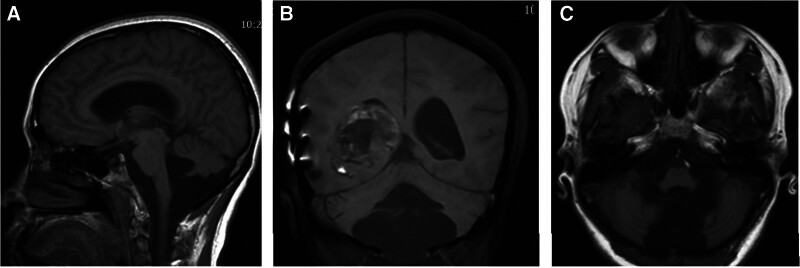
Posterior fossa MRI sections showing Dandy-Walker variant. Imaging of the posterior fossa showing increased retrocerebellar cerebrospinal fluid in the sagittal plane (A), cerebellar vermis hypoplasia with lateral ventricle mass in the coronal plane (B), and enlargement of the cerebellomedullary cistern and the fourth ventricle (A and C). The height of the torcula is normal (A).
